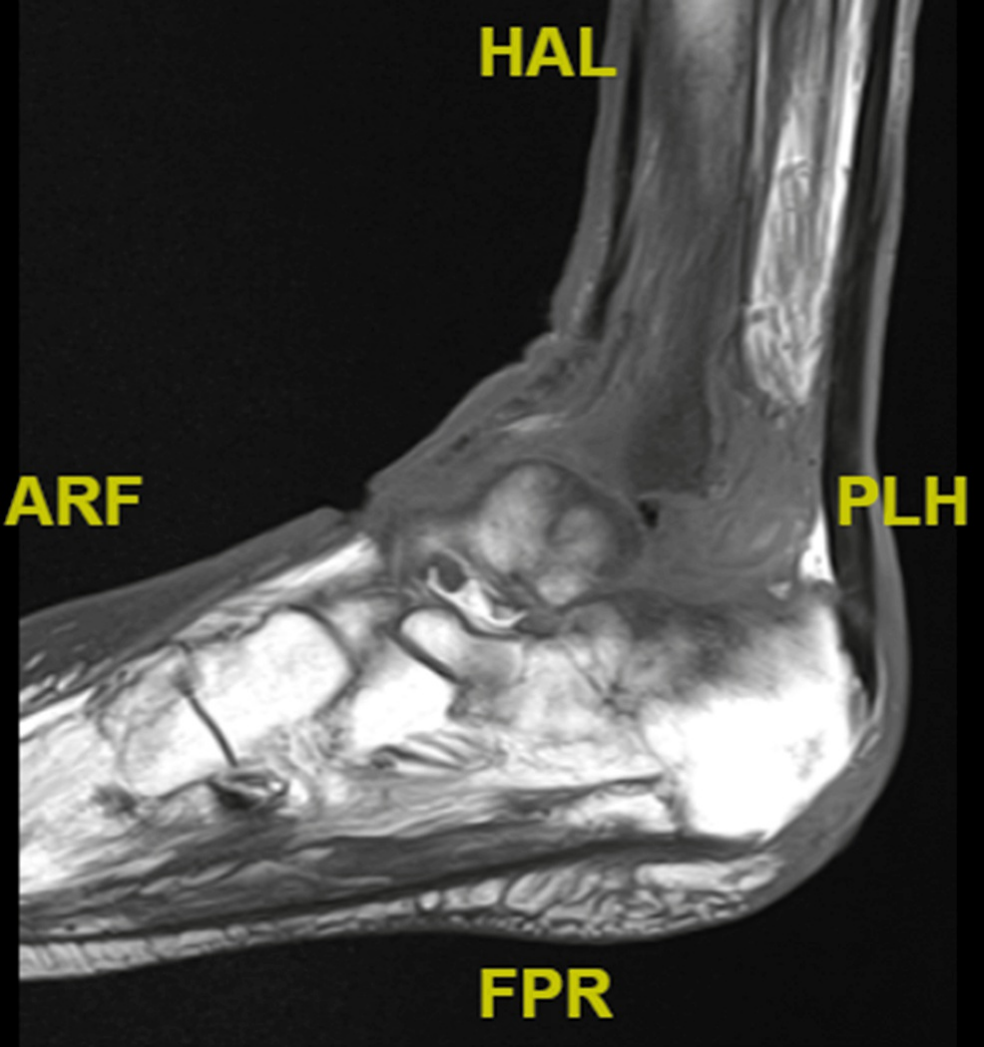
MRI without contrast of the left ankle/foot HAL: high ankle ligament, ARF: anterior radial fossa, PLH: posterior lateral heel.
